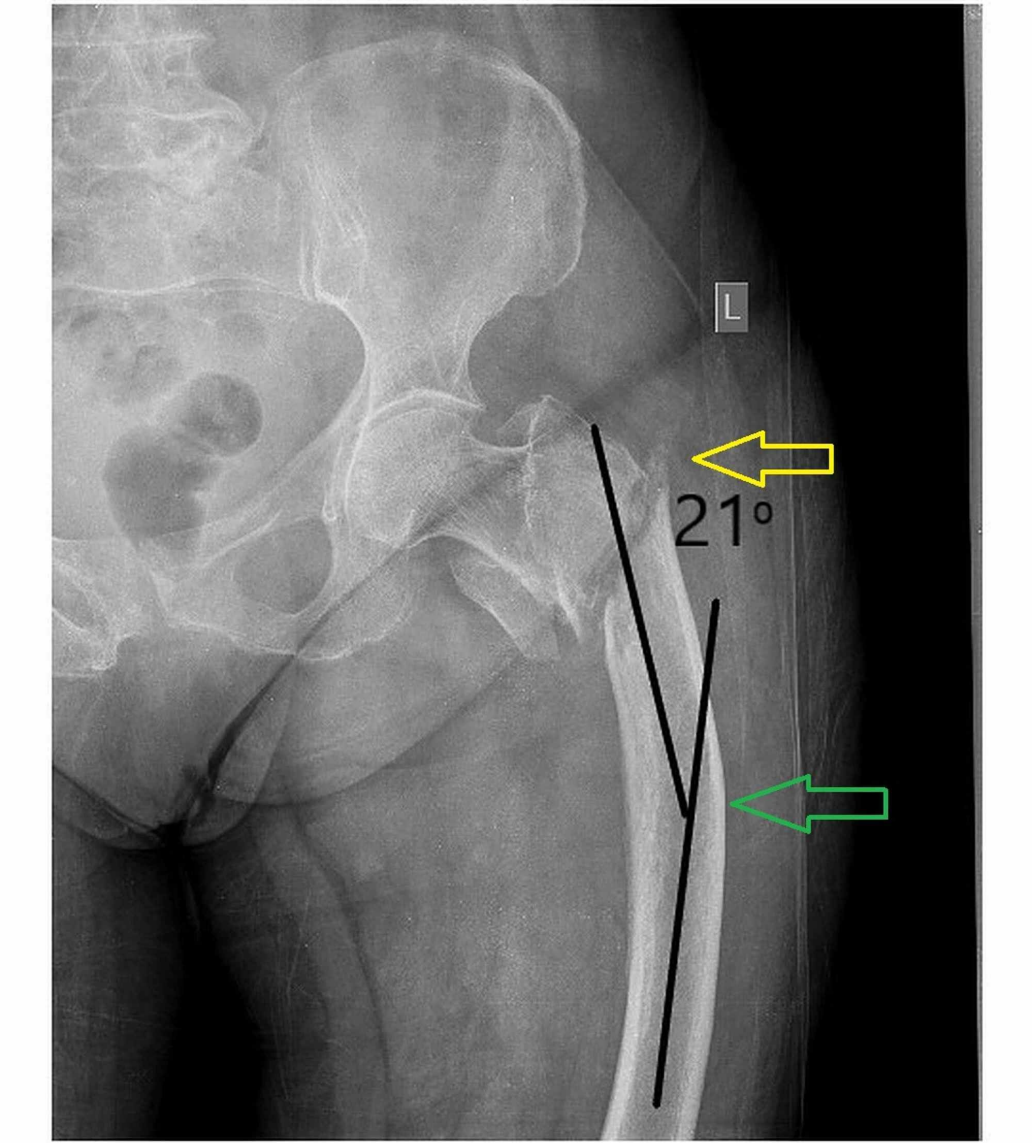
Anteroposterior x-ray of hip and proximal femur demonstrates intertrochanteric fracture and excessive lateral femoral bowing (21 degree). The yellow arrow shows the hip fracture, while the green arrow shows the femoral bowing.
